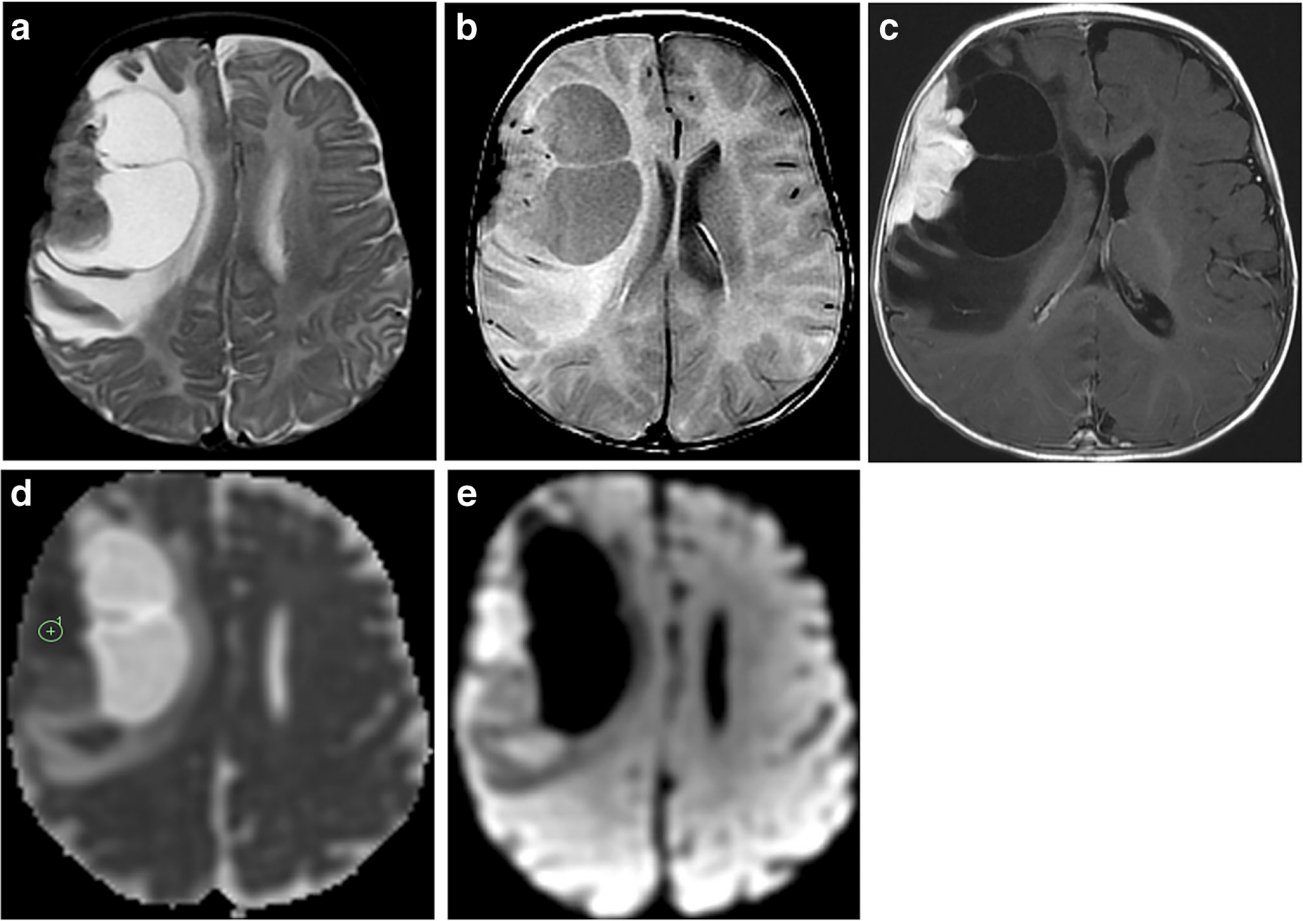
Desmoplastic infantile ganglioglioma. a Axial T2-weighted scan through the frontal lobes shows a large, peripheral, cystic tumor that has a large mural nodule at the leptomeningeal surface of the right frontal lobe. The nodule is hypointense compared to the adjacent gray matter. Moderate T2 abnormality extending well beyond the tumor margin that is also visible on axial T2 FLAIR image (b). c The post-contrast T1-weighted image demonstrates intense enhancement of the mural nodule that is attached to the leptomeningeal surface, a characteristic finding in this tumor. d The ADC map shows heterogeneous but low ADC values within the nodule (ADC_min_=497 × 10^−6^ mm^2^/s) and high value over the cystic component. e The DWI image demonstrates hyperintensity at the corresponding area of ADC_min_
